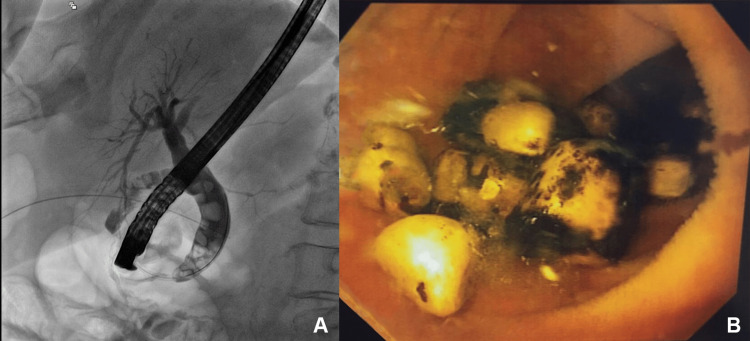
Endoscopic retrograde cholangiopancreatography showing multiple gallstones (A) Abdominal X-ray showing multiple gallstones from the common bile duct to the gallbladder. (B) Multiple gallstones inside the intestinal loop after dilation of the bile duct.
